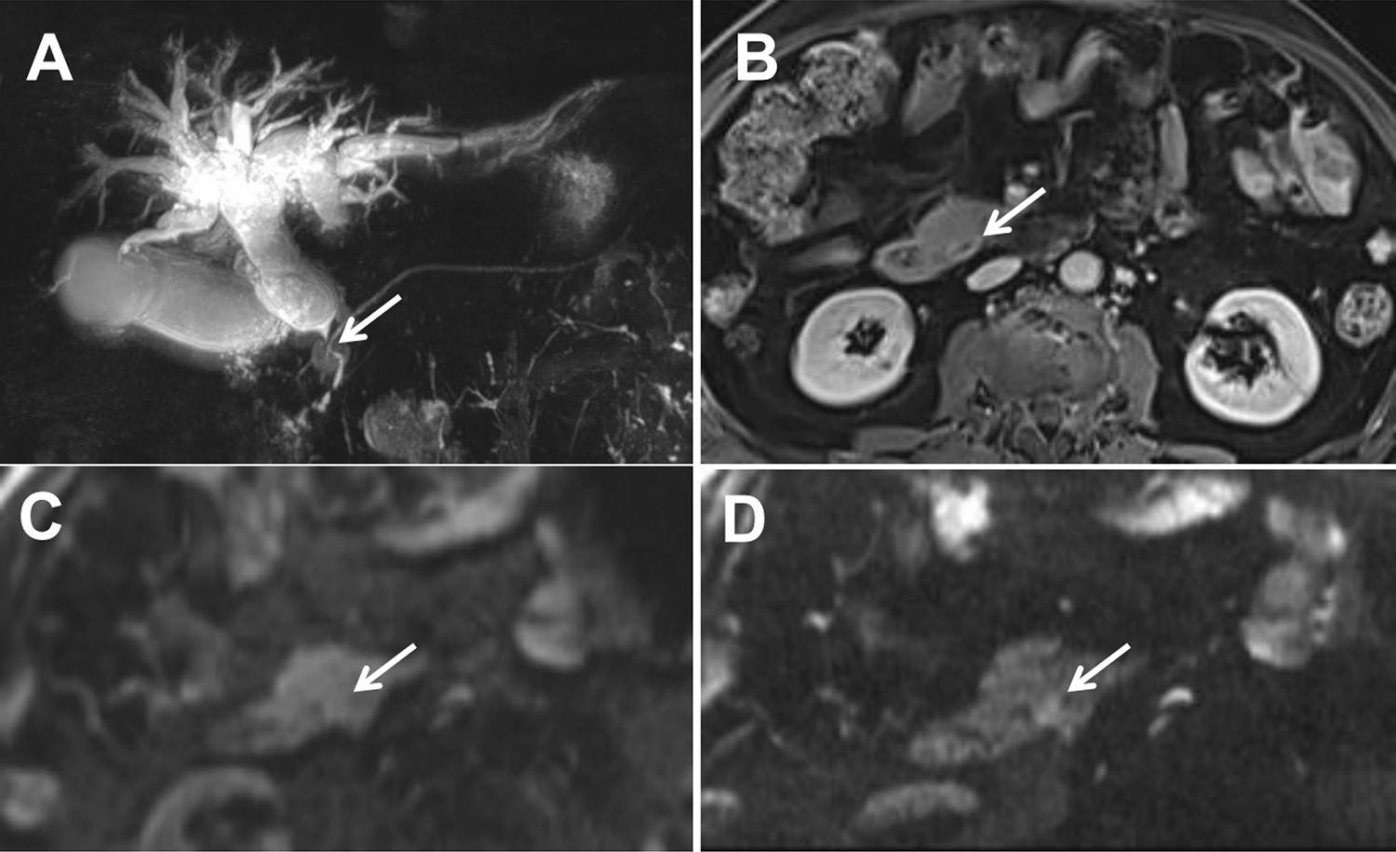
A 72-year-old man with distal common bile duct (CBD) carcinoma with a diameter of 15.3 mm. A MRCP shows marked bile duct dilatation with stenosis of the distal common bile duct (CBD) carcinoma. Main pancreatic duct dilatation was not noted. B T1-weighted MR delay phase image shows the thickened wall of the distal CBD with obvious enhancement. C c-EPI DWI shows no abnormality in the periampullary region. D z-EPI DWI shows increased signal intensity of the lesion with better delineation
